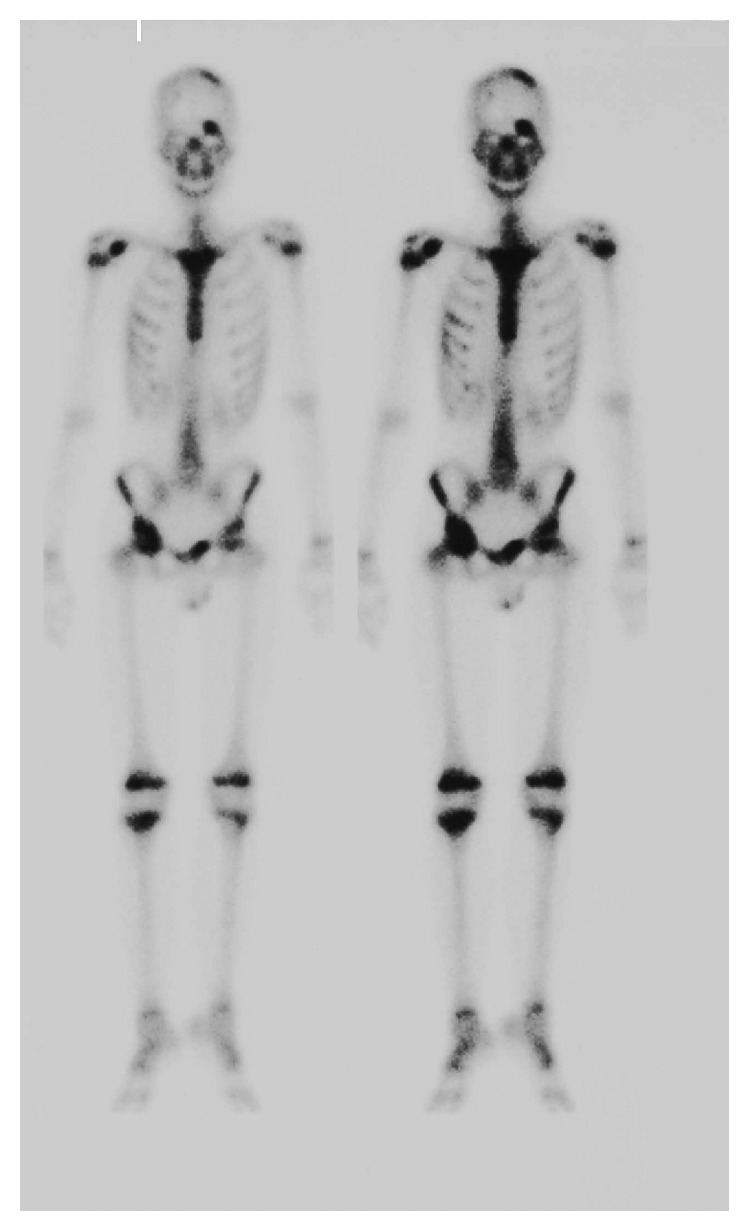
Bone scintigraphy showing multiple lesions in the left frontal bone, sternum, right superior supraclavicular fossa, and iliac bone, suggesting fractures, inflammation, or malignant cells.
